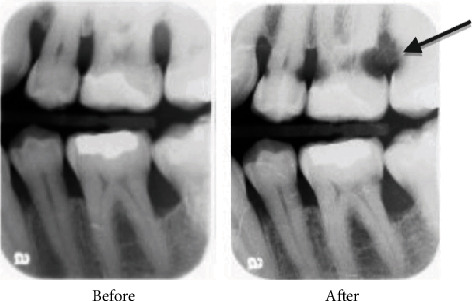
Vertical bitewing radiographs of the left maxillary and mandibular first molars. The “before” was taken November 20, 2006. The “after” was taken December 1, 2009, 19 months after the patient started using nicotine lozenges. Note the extensive root caries involving the mesial and distal surfaces of the maxillary teeth and distal of the mandibular first molar.
